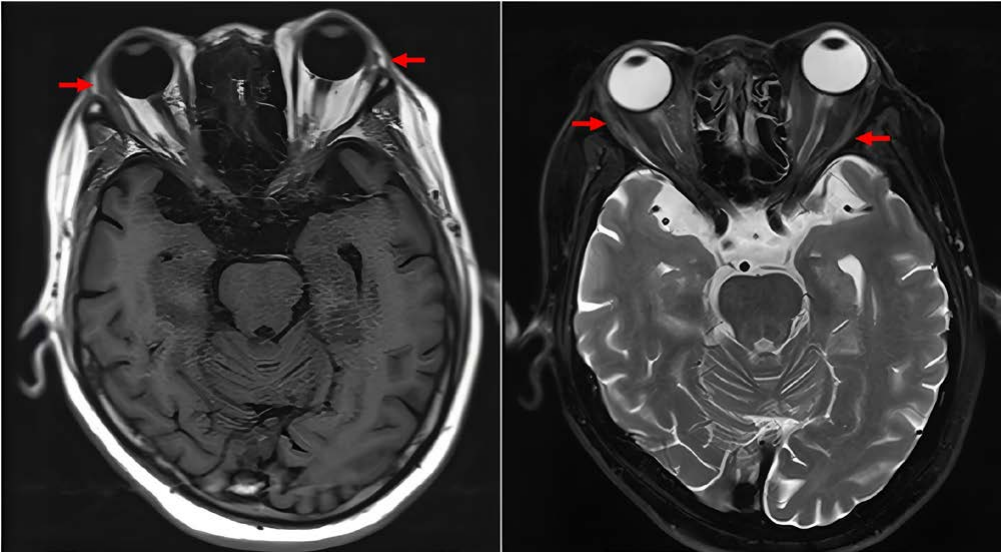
Plain + contrast-enhanced orbital MRI: size reduction was observed in certain lesions.
